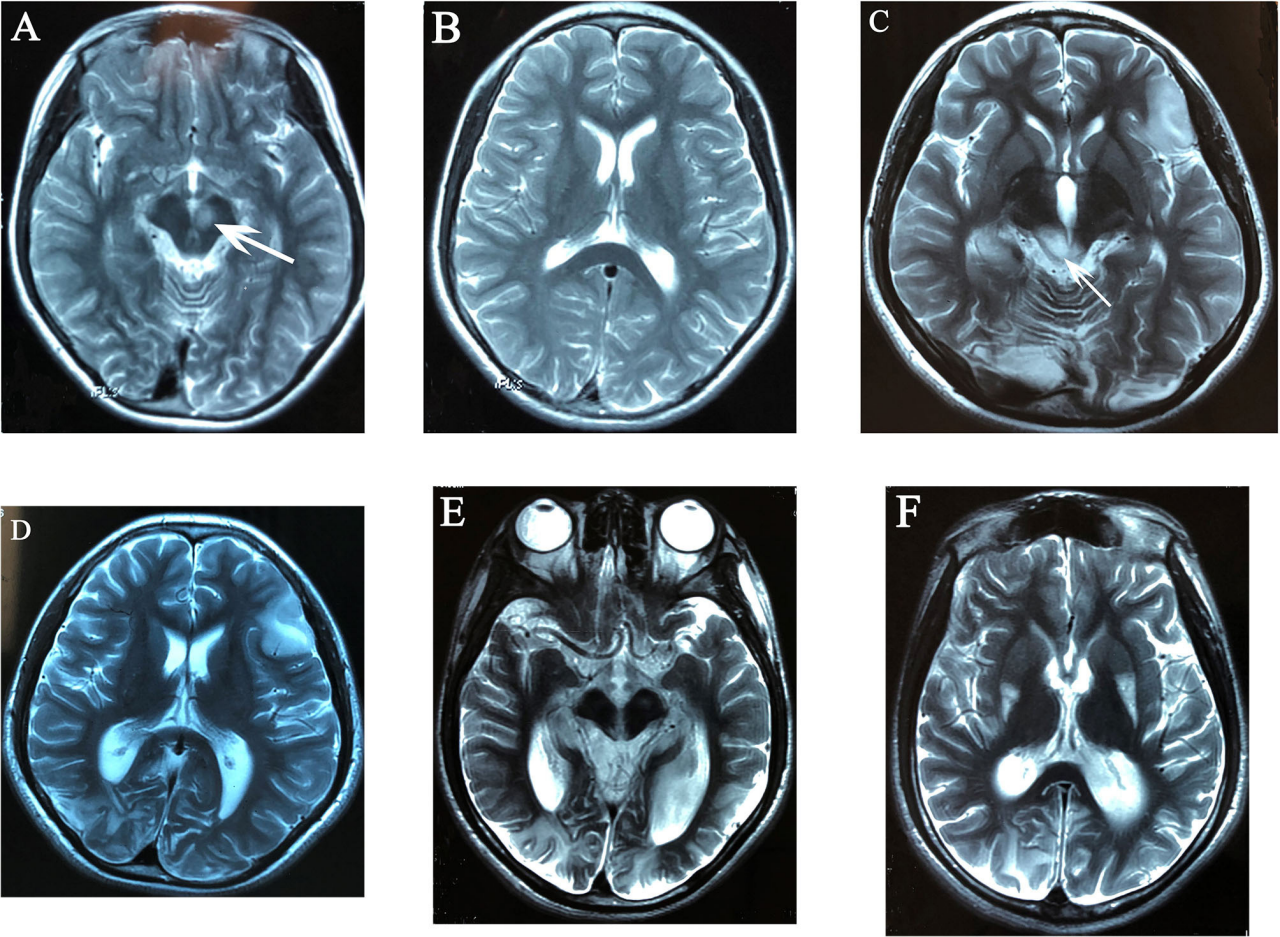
Evolutionary changes in T2-weighted MR images of case 4: high signal intensity of left red nucleus (A), no cortical lesions at that time (B). After 2 years, hyperintense lesion of bilateral occipital lobes, right temporal lobe, and left frontal lobe centered in the cortex and subcortical white matter (C,D), high signal intensity of bilateral superior and inferior colliculi, right lesions were more severe than left sides (C), mildly enlarged bilateral occipital horns of lateral ventricles (D); 6 years after onset, the brain atrophy was progressive with occipital lobes being predominant, the enlargement of bilateral occipital horns of lateral ventricles was more significant (E,F), and high signal intensity over posterolateral 1/3 of bilateral putamen was found (F).
